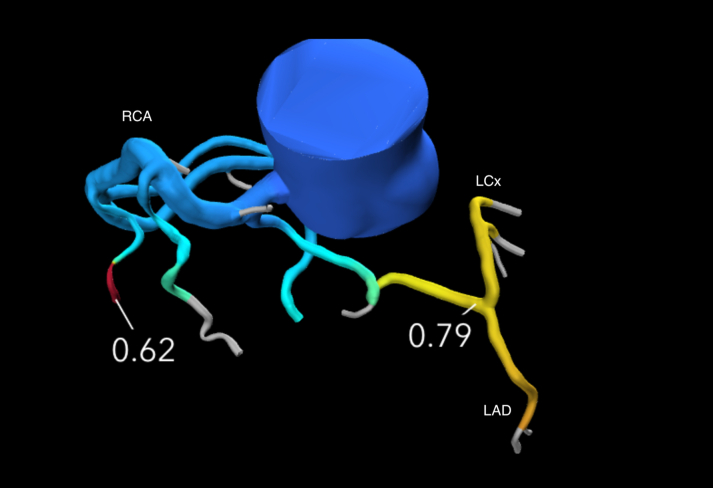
CT-FFR Analysis Demonstrated a Drop in Fractional Flow Reserve Distal to the “Curly-Q” Kink in the Anomalous Left Anterior Descending Artery, Indicating Physiologic Significance of the Lesion
